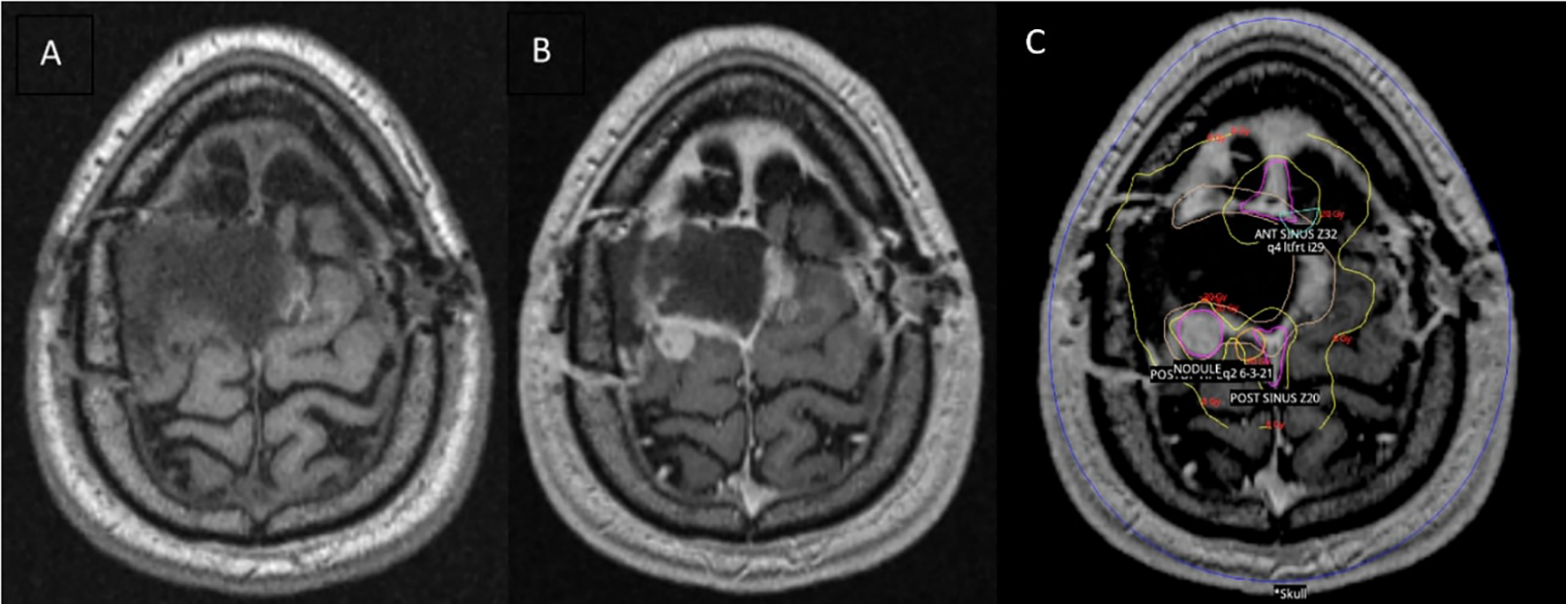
Brain MRI performed 44 days post-operatively and overlying radiotherapy treatment plan. (A) MRI T1 pre-contrast. (B) MRI T1 post-contrast showing peripheral nodular enhancement on the left lateral and posterior surgical bed. (C) Gamma Knife (GK) radiosurgery treatment plan overlying T1 post-contrast MRI brain. The wide post-operative area is represented by the yellow contour. The pink contours delineate the three nodular, high-risk areas.
